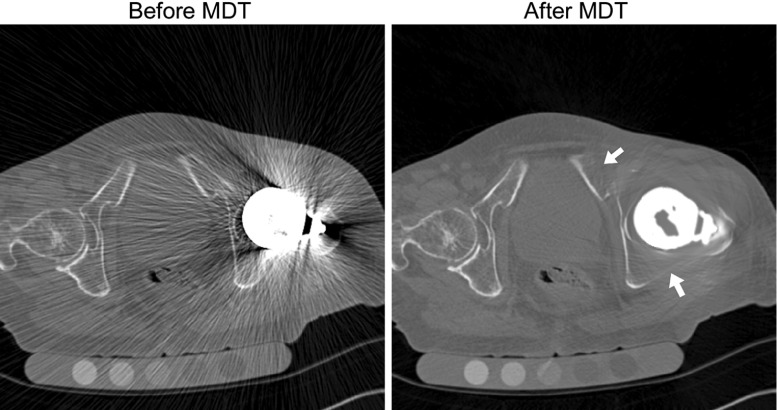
Axial CT slice with an Austin Moore hip implant before and after MDT, with the arrows indicating the fading or complete disappearance of the cortex
